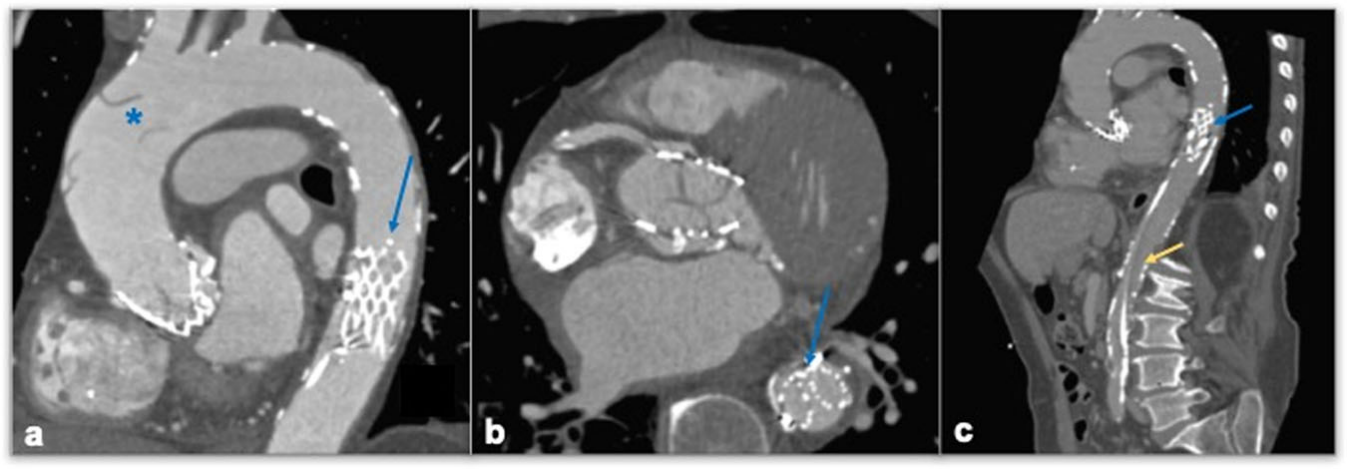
A 55-year-old patient CTA control after TAVI positioning for prosthesis dislocation. CTA images show, aortic prosthesis dislocation in the descending aorta (a, b, c blue arrow), causing focal ascending aorta dissection (blue asterisk). Sagittal MPR also shows the presence of a vascular catheter inside the thoracoabdominal aorta, trapped in the dislocated prosthesis (c, yellow arrow)
